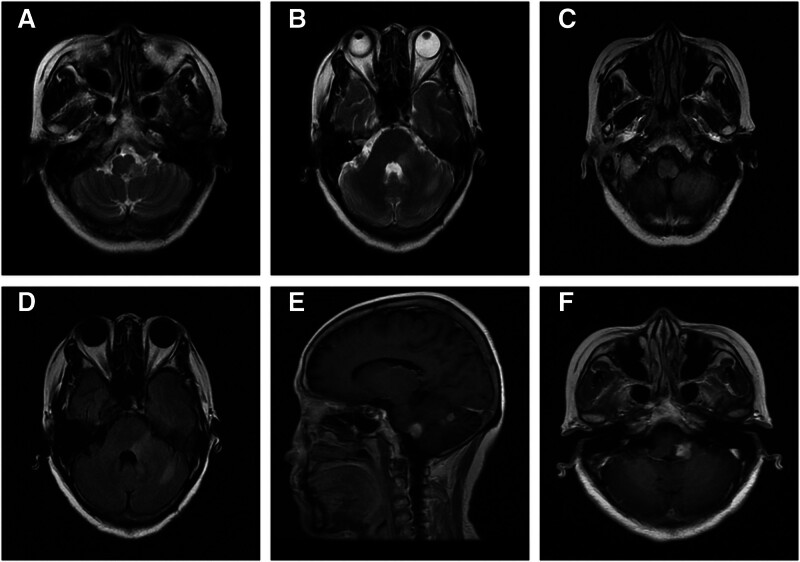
Initial admission cranial MRI. The left pons and cerebellar hemispheres exhibited slightly elevated lesion signals on both T1WI and T2WI (A–D). The enhanced MRI of the head revealed nodular enhancement of the lesions located in the left pontine region and cerebellum (E, F). MRI = magnetic resonance imaging, T1WI = T1-weighted imaging, T2WI = T2-weighted imaging.
